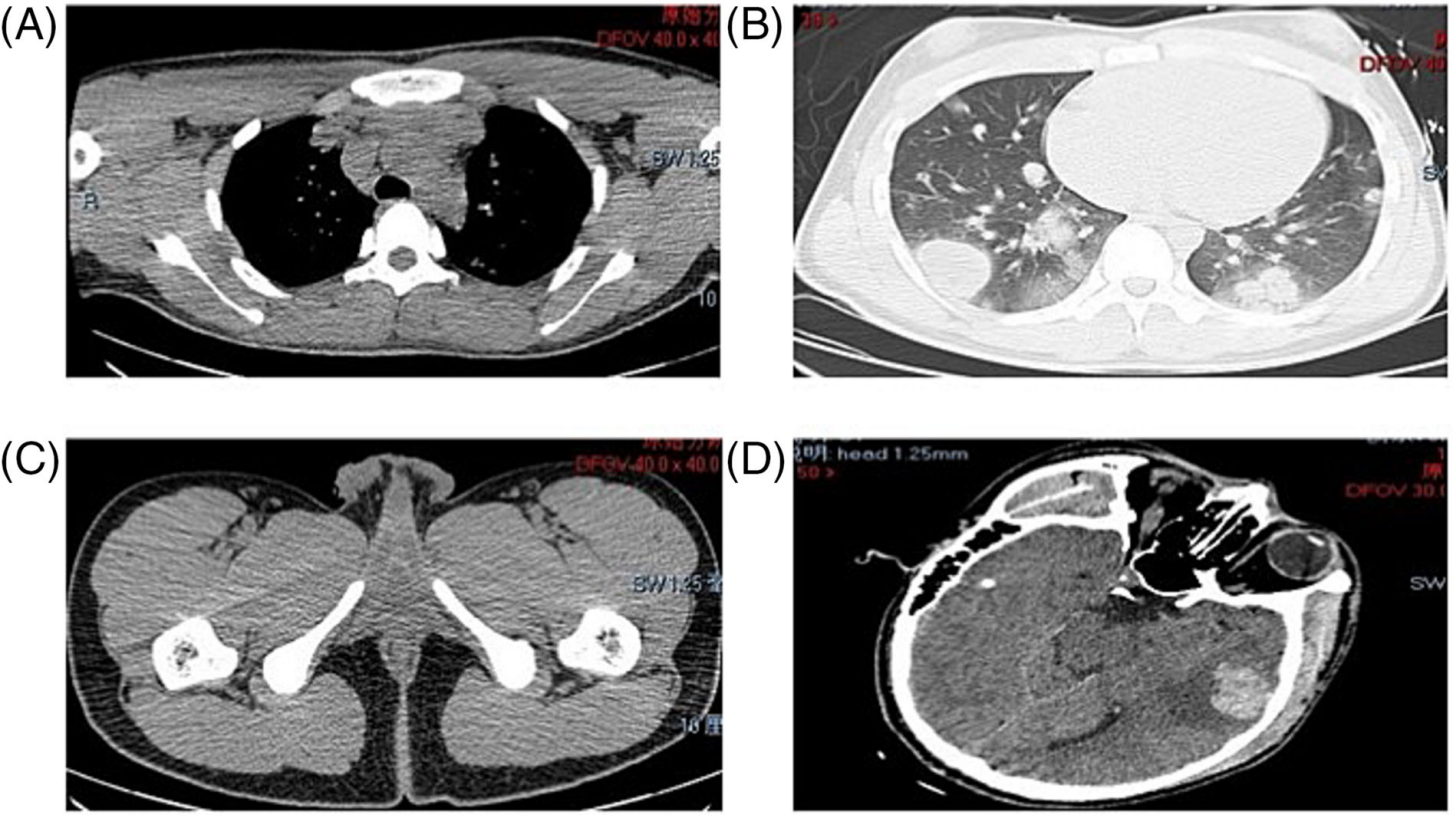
Total body CT scan. (A) A mediastinal window image revealed a heterogeneous tumor. (B) A pulmonary window image showed masses in the both lung lobe. (C) Pelvis CT showed no abnormal mass shadow in the bladder or gonads. (D) Left frontotemporal brain hemorrhage.
